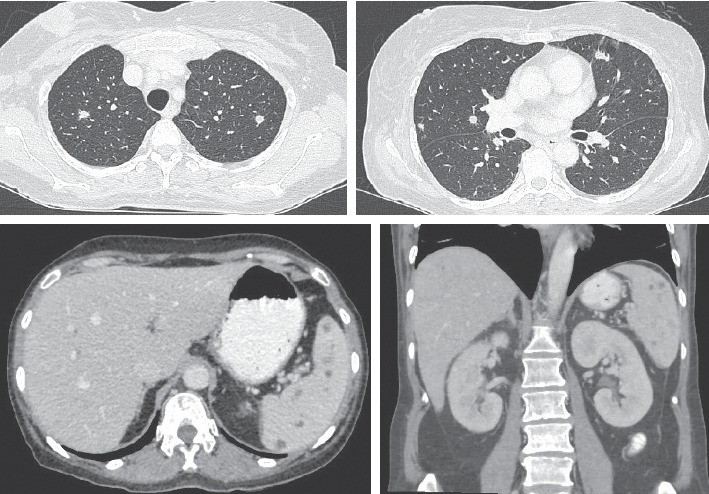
CT revealed multiple irregular small discrete nodules in both lungs size <1 cm and multiple nonenhanced hypoechoic nodules in the liver and spleen, microabscesses.
